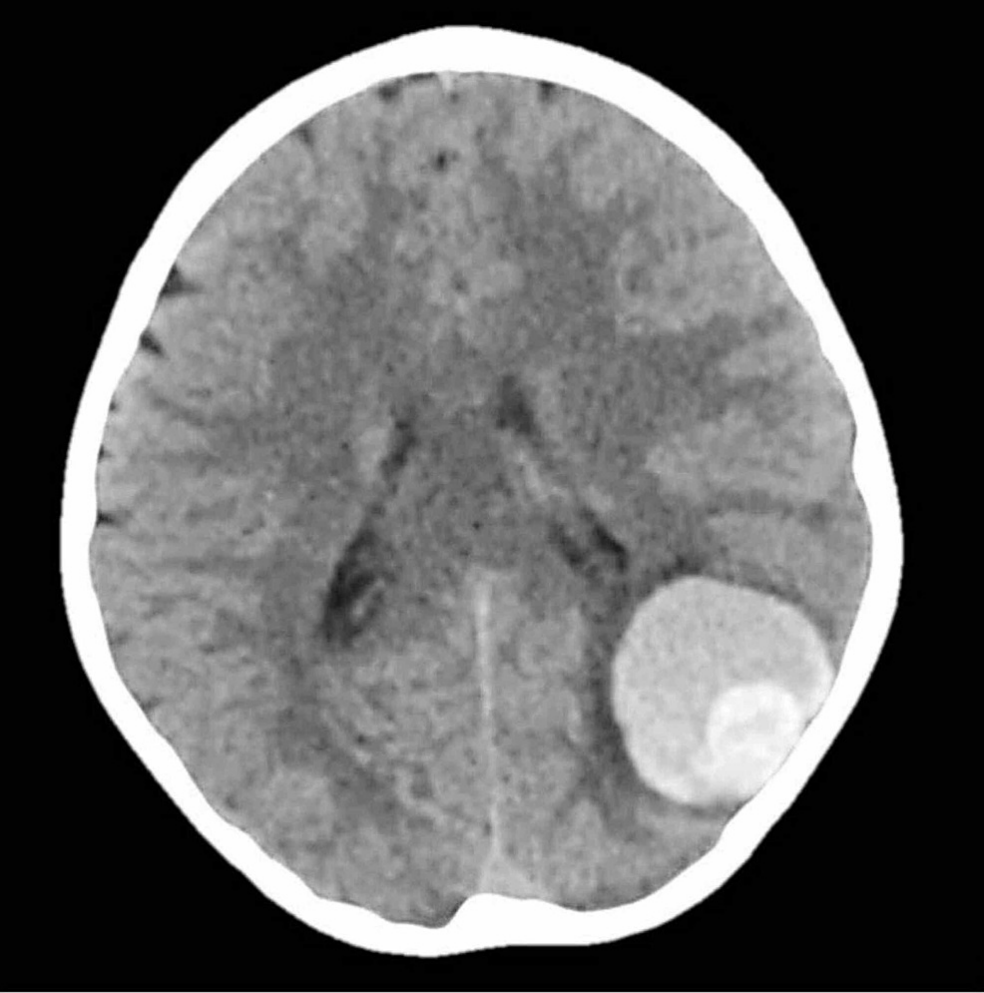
Axial brain CT scan. Axial brain CT scan on admission showing a voluminous hemorrhage in the left temporo-occipital area.
